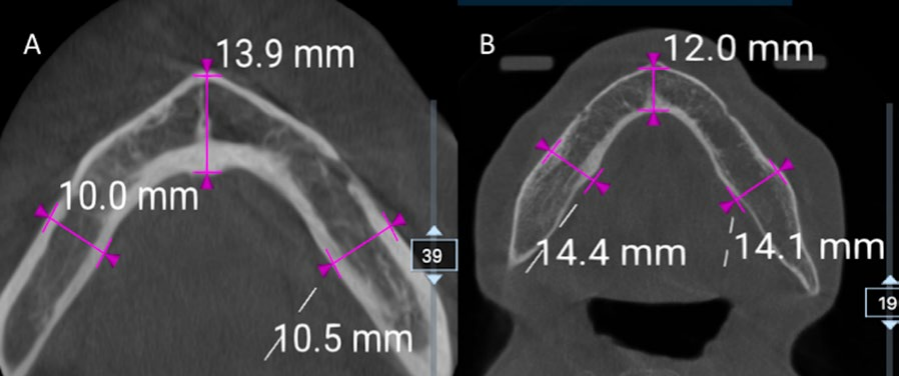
Axial CBCT images of two different patients (A and B) with mandibular bone thickness measured in the symphysis and in both hemiarches at the level of the first molars
